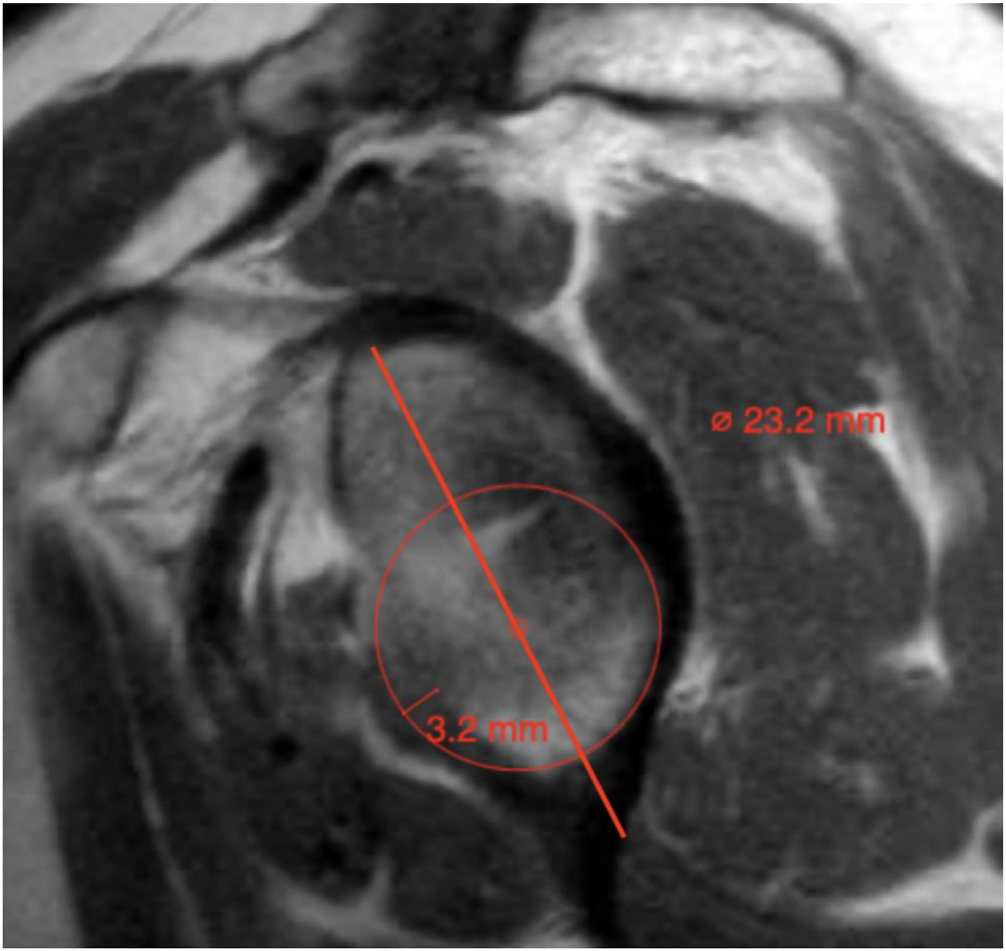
Sagittal view at glenoid face of left shoulder showing 3.2 mm of glenoid bone loss using perfect circle method on magnetic resonance imaging. (Ø, circle diameter.)
